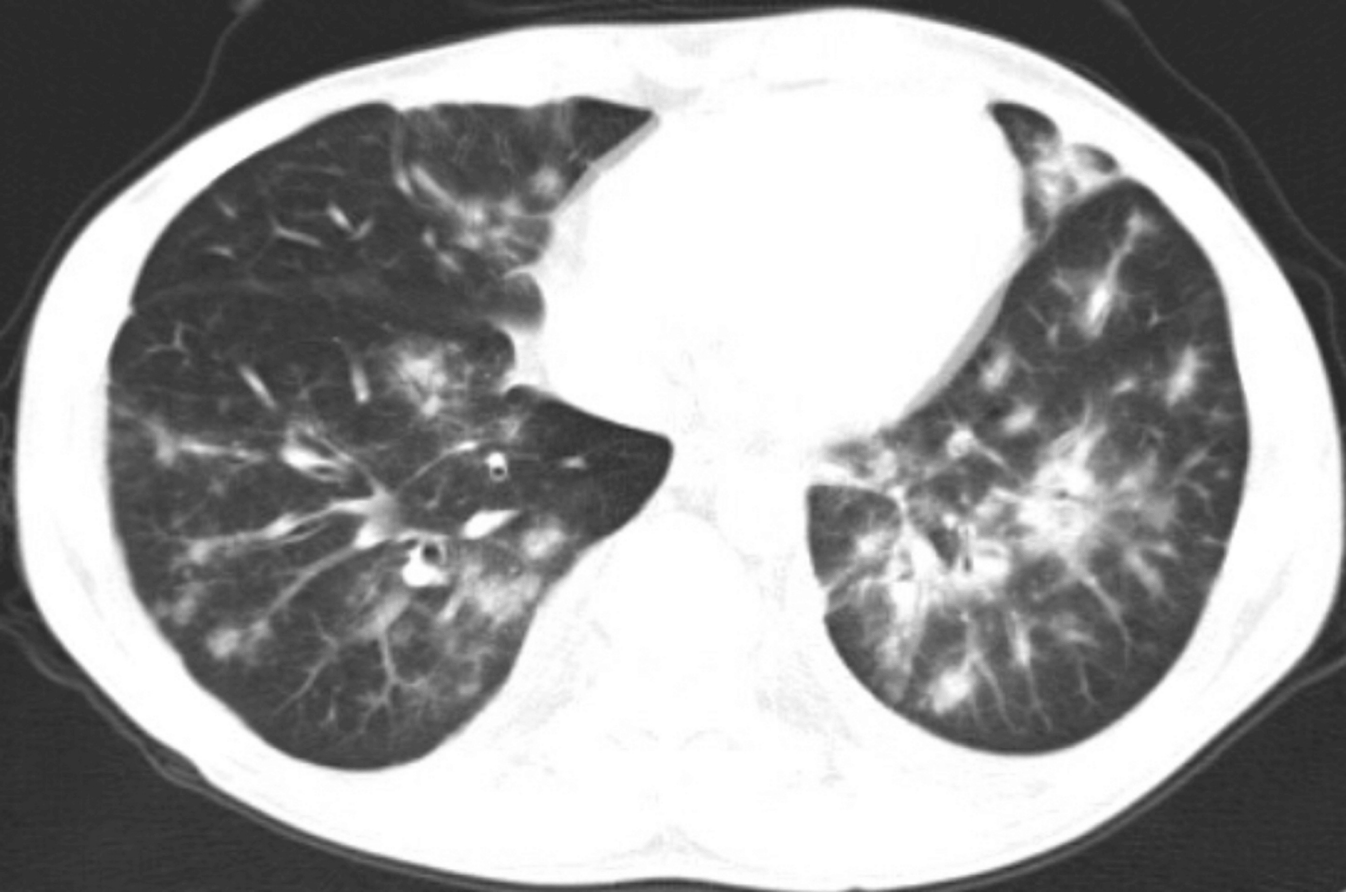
CT scan of the chest showing diffuse bilateral reticulo-nodular opacities and infiltrates, a characteristic of pulmonary Kaposi sarcoma
